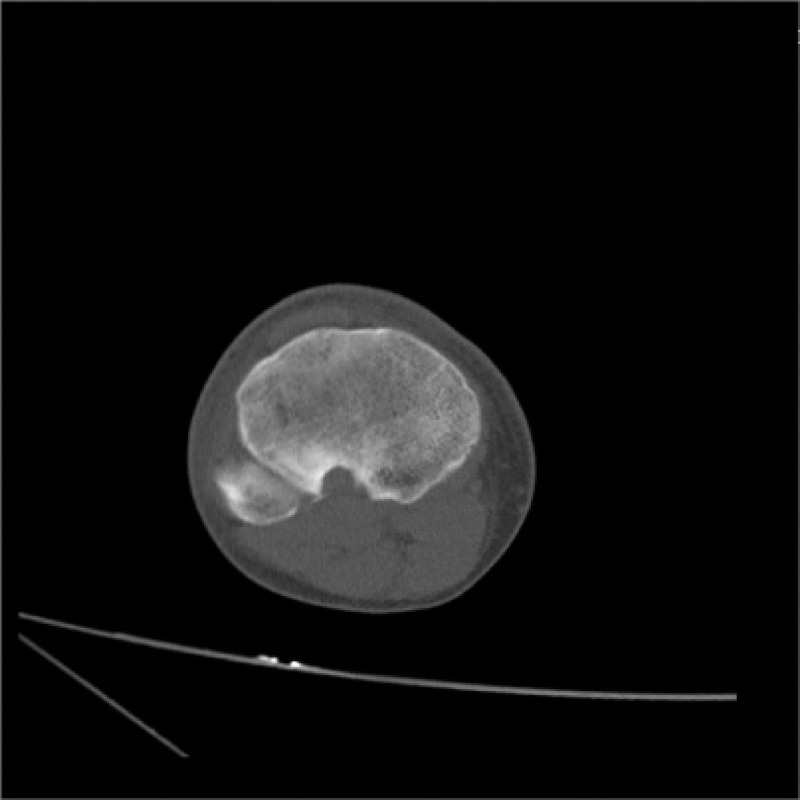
Postoperative CT image in transverse plane. CT = computed tomography.
